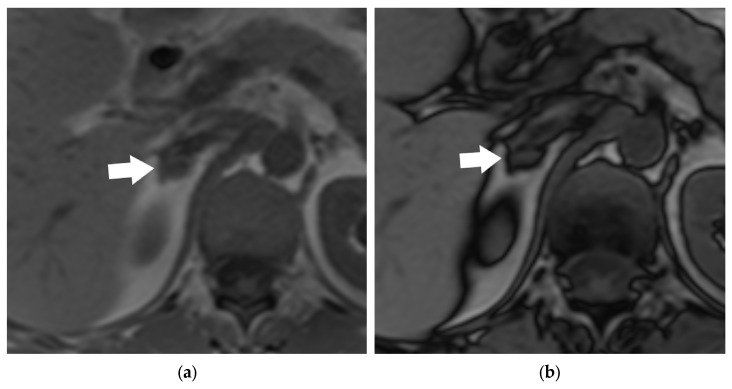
Chemical-shift MRI characterization of adrenal mass: Axial in-phase T1-weighted MRI image showing a mass (arrow) in the right adrenal gland (a). There is no signal loss of the mass (arrow) on the out-of-phase T1-weighted image (b), suggesting an adrenal metastasis over an adrenal adenoma in a patient with lung cancer.
